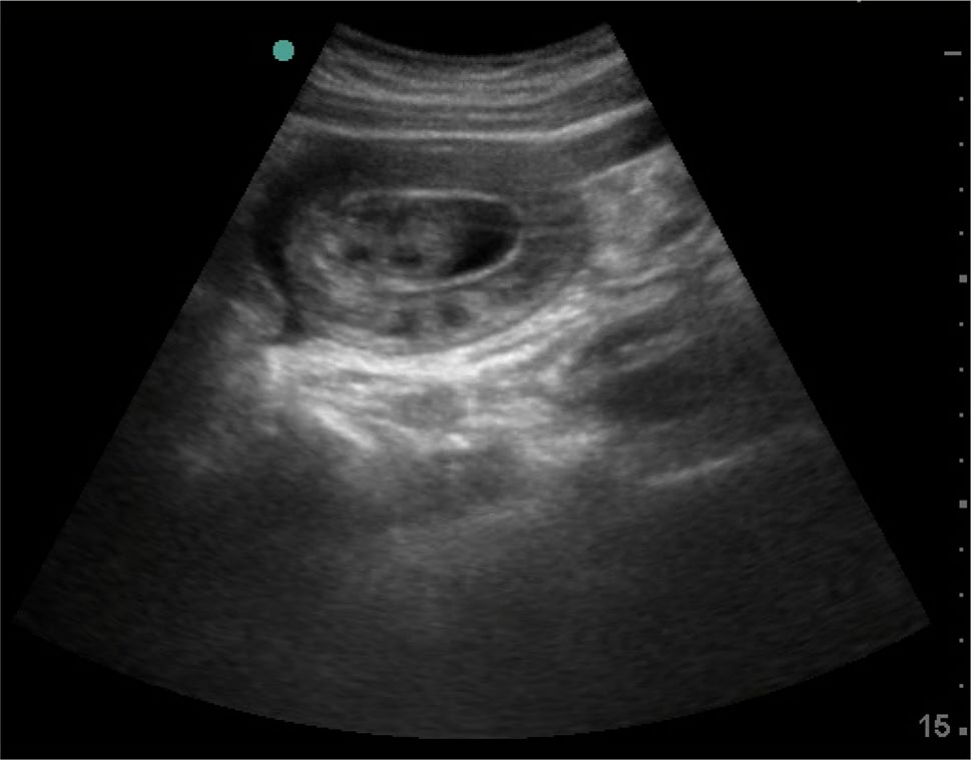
Point of care ultrasound for acute abdomen (ACUTE approach) showed localized free fluid with doughnut sign edematous bowel loop wall
